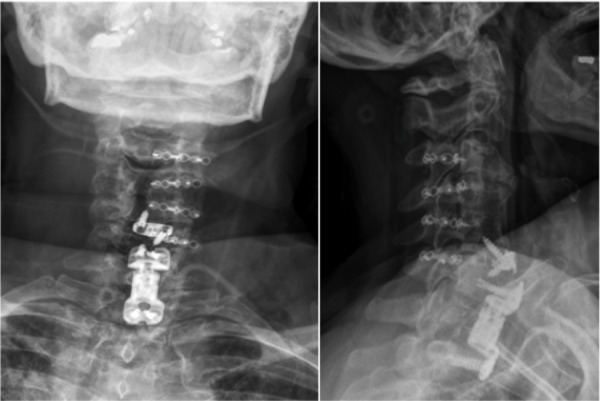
Anterior-posterior (AP) and lateral postoperative x-ray demonstrating new and old hardware with no signs of fracture or displacement
